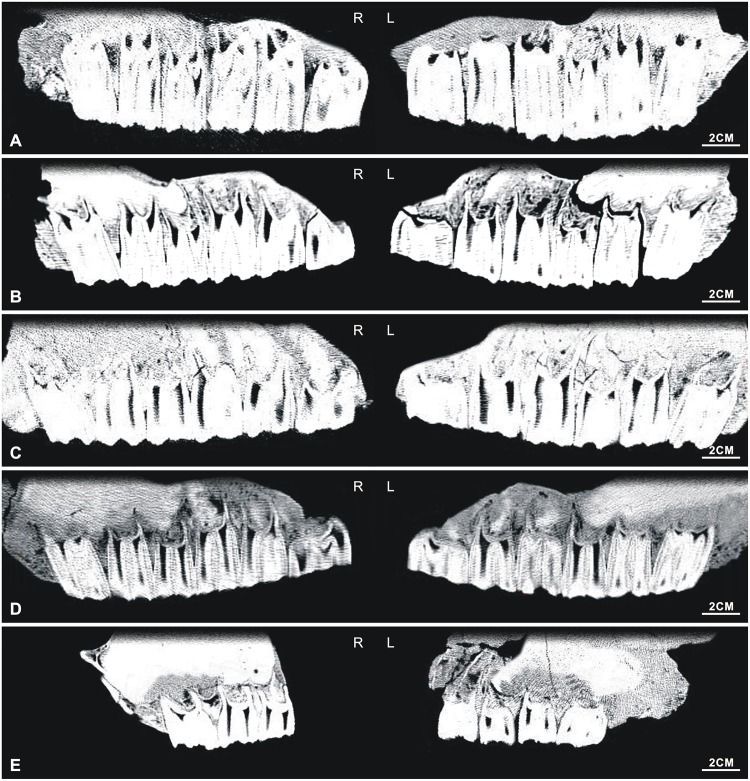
CT reconstruction images of the inlaid maxillary cheek teeth. (A) NWU1420; (B) NWU1421; (C) NWU1419; (D) NWU1425; (E) NWU1423.
